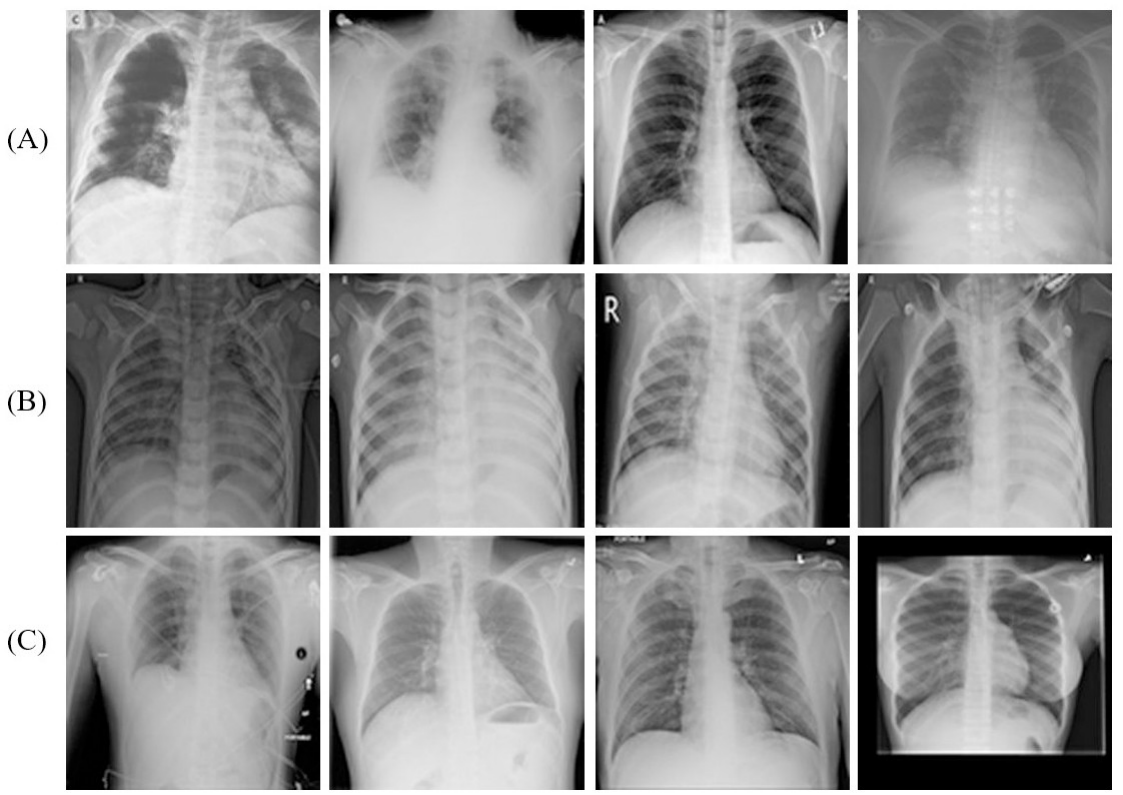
Some cases of CXR from the COVID-19 Radiography Database. (A) COVID-19 sample, (B) Pneumonia sample, and (C) Normal sample.
